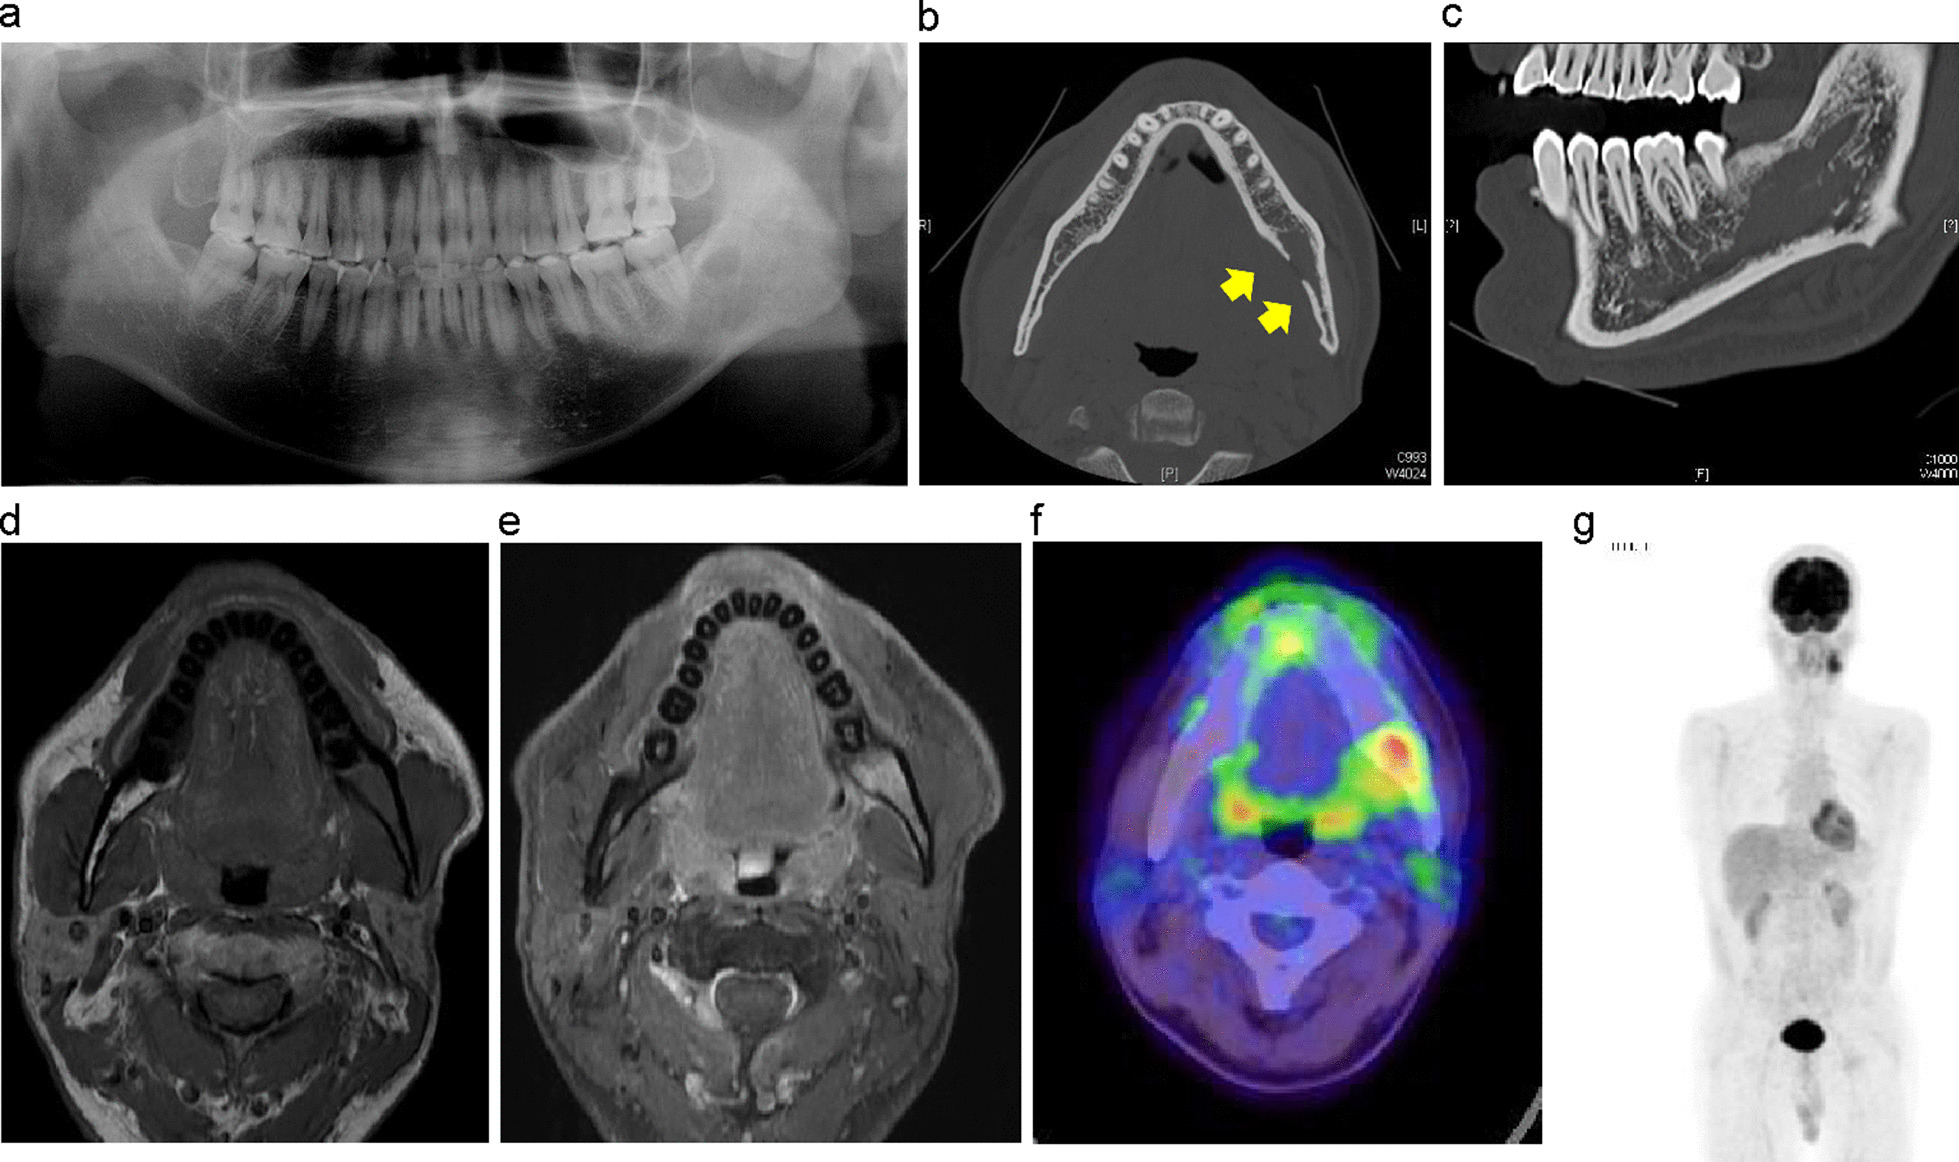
Case 1. A 45-year-old man with DLBCL, stage IA. a Panoramic radiograph image taken at the first visit showing a radiopaque area in the mandibular left third molar area. b Axial CT scan using a bone algorithm at the first visit showing penetrating resorption of the cortical bone in the lingual aspect of the left mandible (yellow arrows). c Sagittal CT scan image showing marginal resorption of the mandibular canal wall. d Contrast-enhanced MRI of the bone marrow of the mandible showed low signal intensity on T1-weighted image. e Homogeneous enhancement on post-contrast fat-suppressed T1-weighted image. f Fluorodeoxyglucose PET/CT scan. g Maximum intensity projection image
